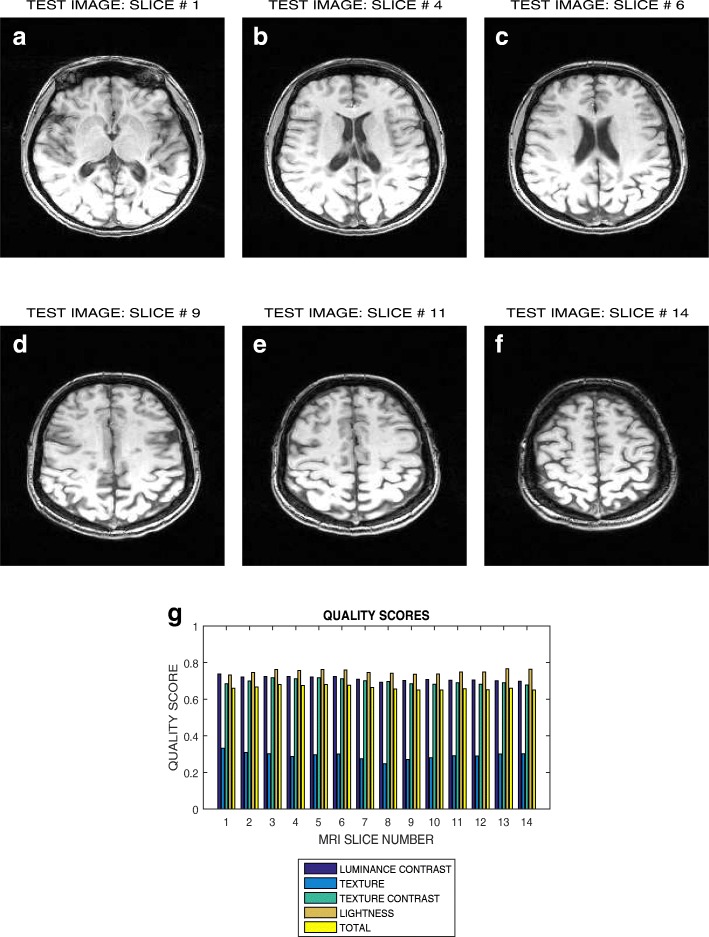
Six slices with indices (a) 1, (b) 4, (c) 6, (d) 9, (e) 11, (f) 14 in a T1 weighted MRI volume data degraded by different configurations of bias fields, (g) luminance contrast, texture, texture contrast, lightness and total quality scores for each slice in the volume data
